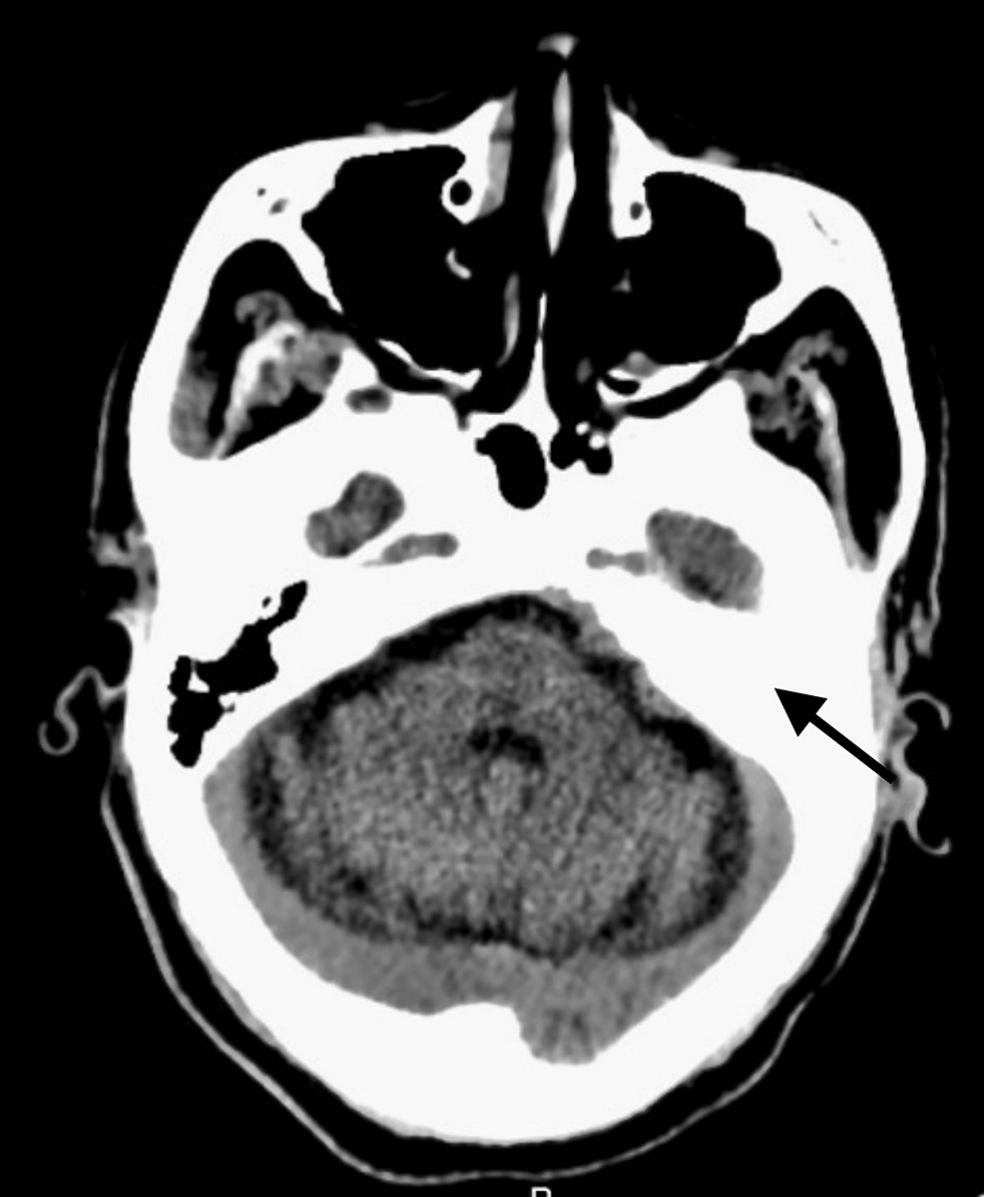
Head CT showing left middle ear and mastoid filling.
